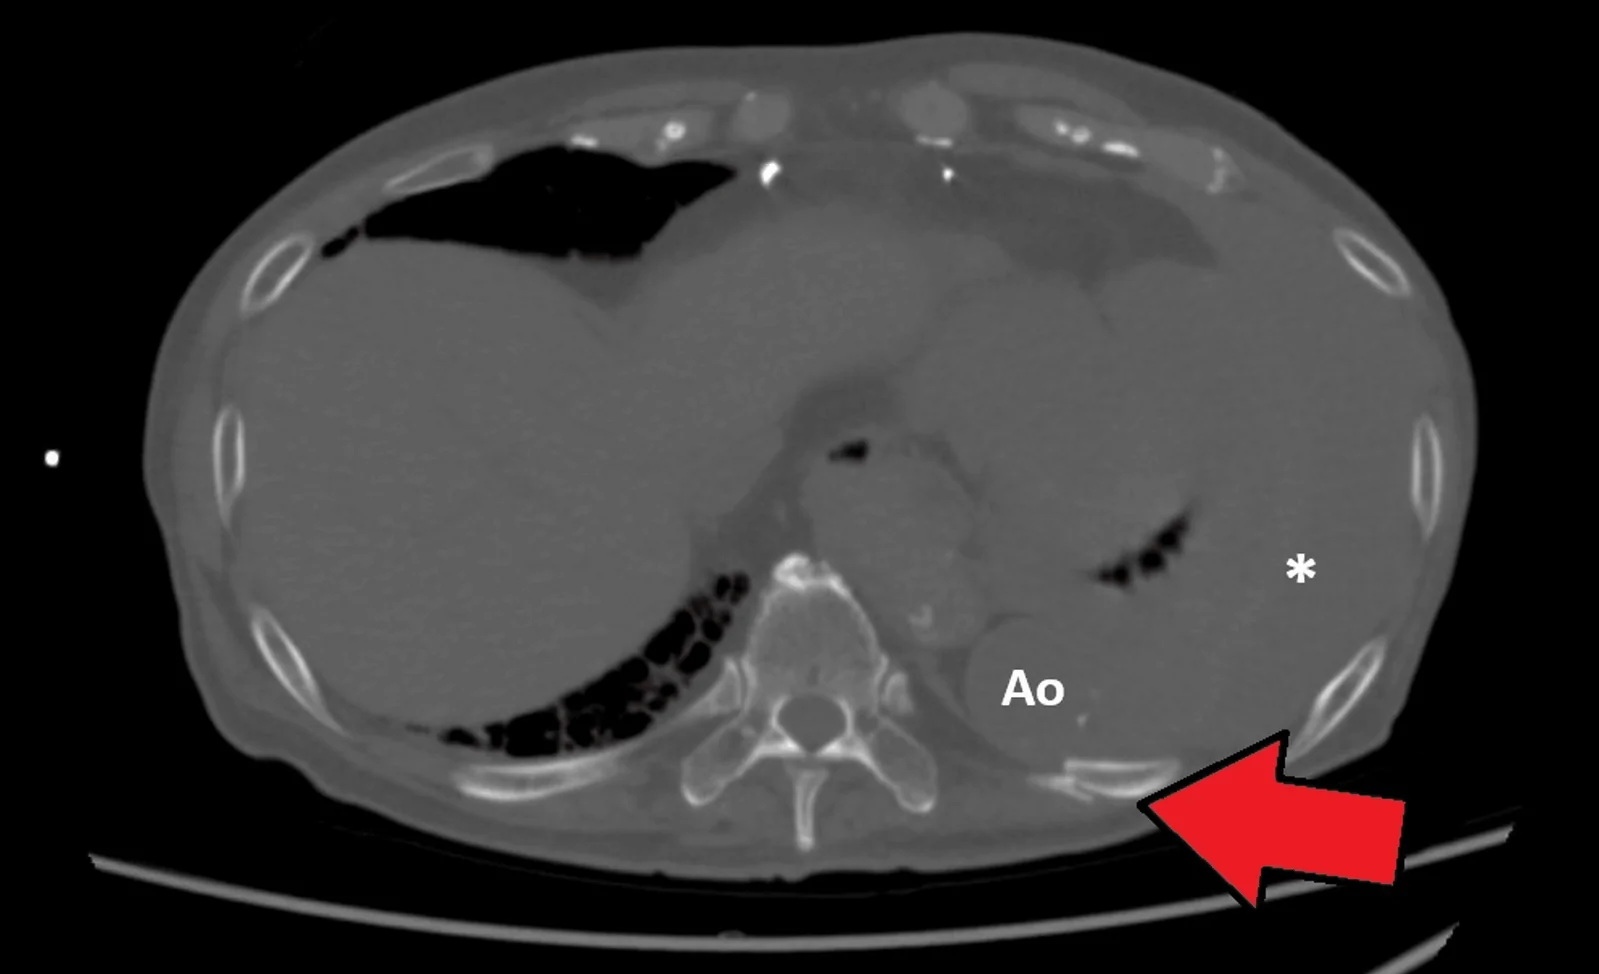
Chest computed tomography obtained after the second fall. Mild displacement of the previously noted left 10th rib fracture is evident, accompanied by a massive left hemothorax. The red arrow indicates the fractured rib, which is located in close proximity to the descending aorta (Ao), and * indicates hemothorax.
